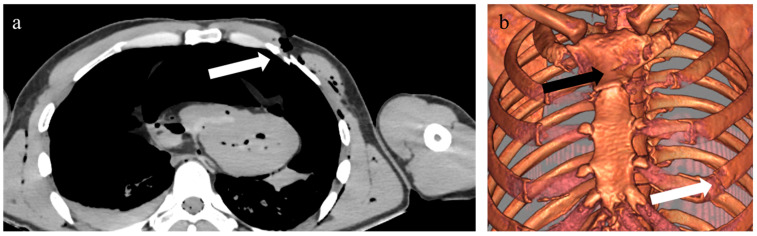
Two-dimensional (a) and three-dimensional (b) view of a sharp force homicide postmortem CT. White arrows indicate the same lesion on the left fourth rib and a sternal lesion (black arrow).
